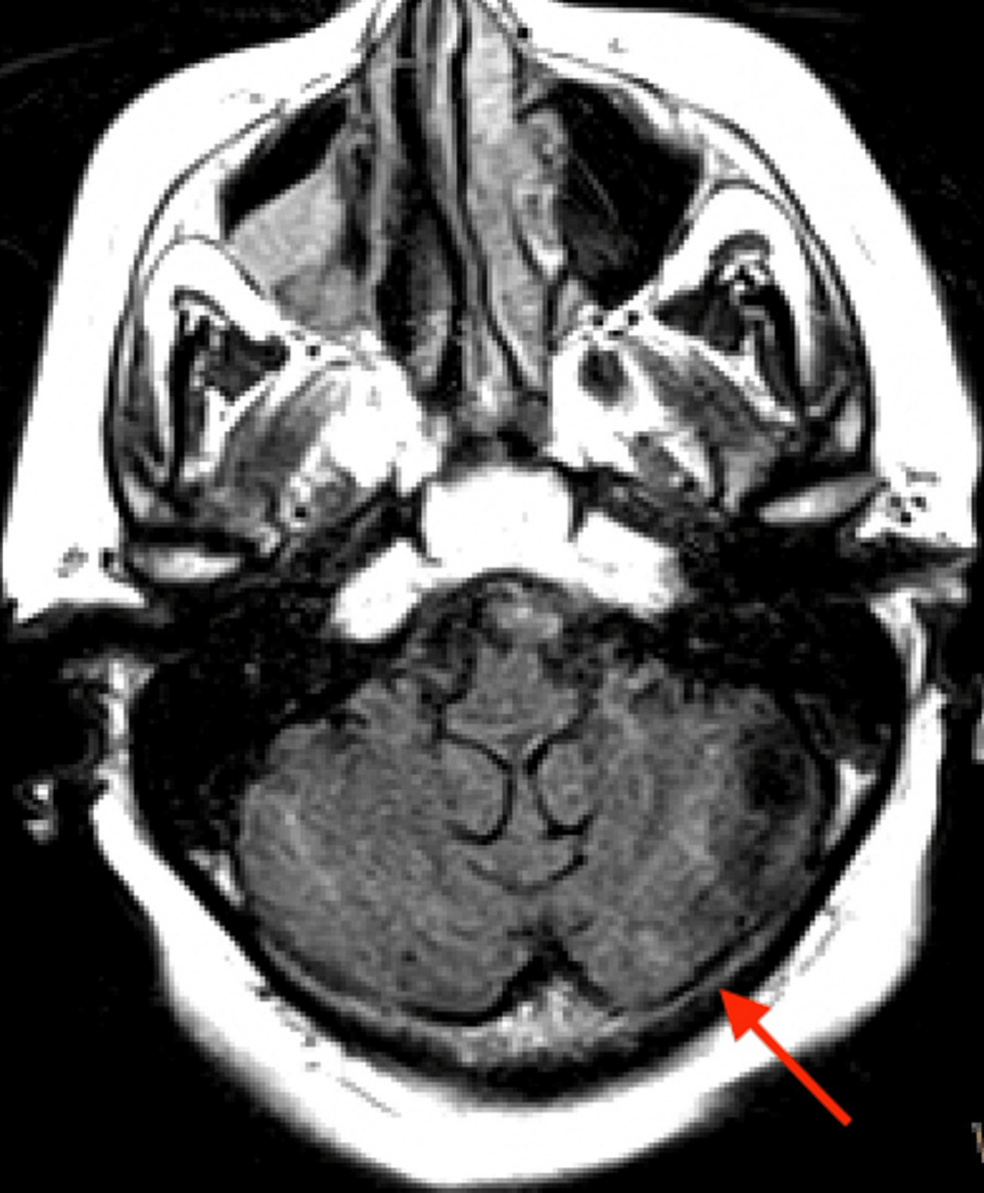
MRI axial flair, acute infarct involving the medial left cerebellar hemisphere.
